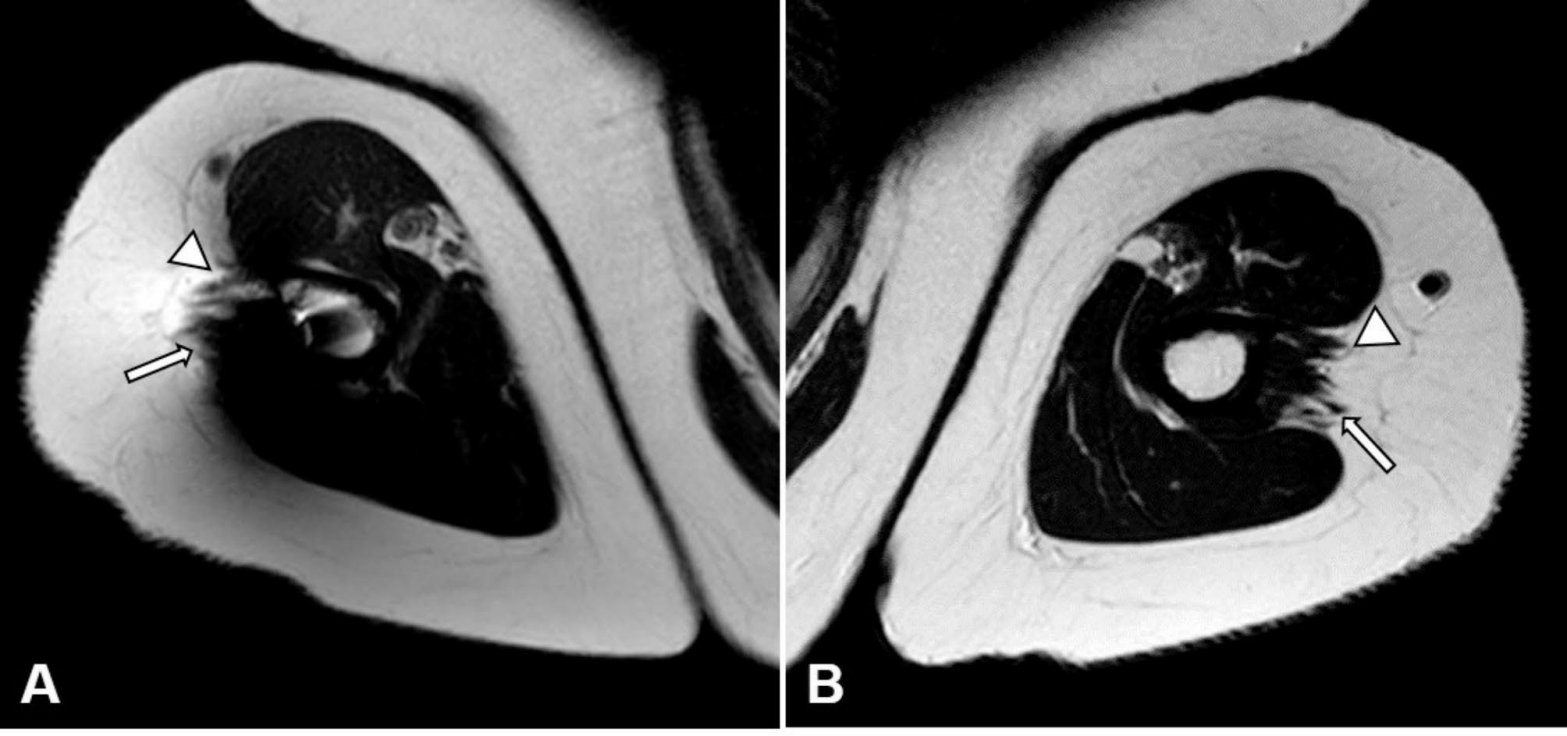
MRI analysis of an operated right humerus (A) and the contralateral side (B) of the same patient as shown in Figs. 1 and 2. The distal deltoid insertion with an intact anterior (triangles) and posterior (arrows) part is demonstrated on these axial images.
